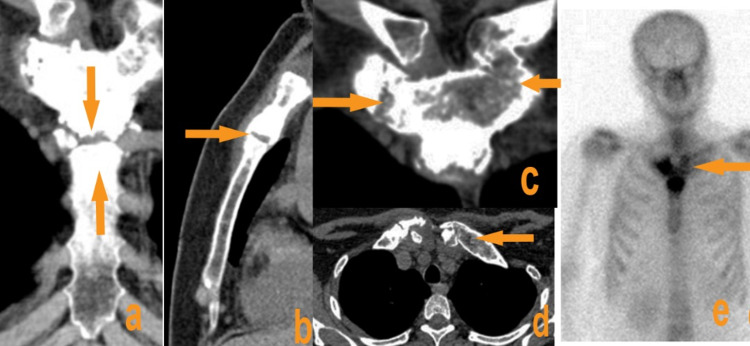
Chest CT scan (a) Coronal and (b) sagittal sections showing chronic osteitis of the manubriosternal joint with ankylosis (arrow). (c) Coronal section: erosion and hyperostosis of the costosternal joints (arrow). (d) Partial fusion of the left sternoclavicular joint (arrow). (e) Hyperfixation of the anterior chest wall on whole-body scintigraphy shows a bull's horn appearance.​​​​​​​
